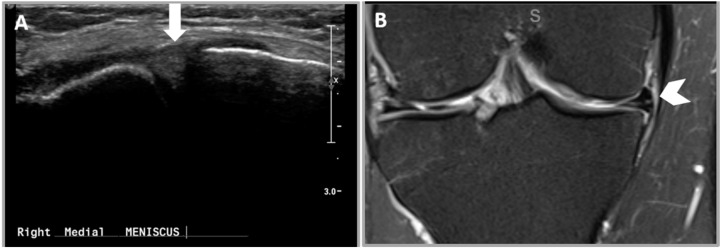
False negative medial meniscal tear in US. (A) US image demonstrating normal medial meniscus (white arrow). (B) A coronal proton density MRI image of the right knee demonstrating a horizontal tear of the medial meniscal body (white chevron).
